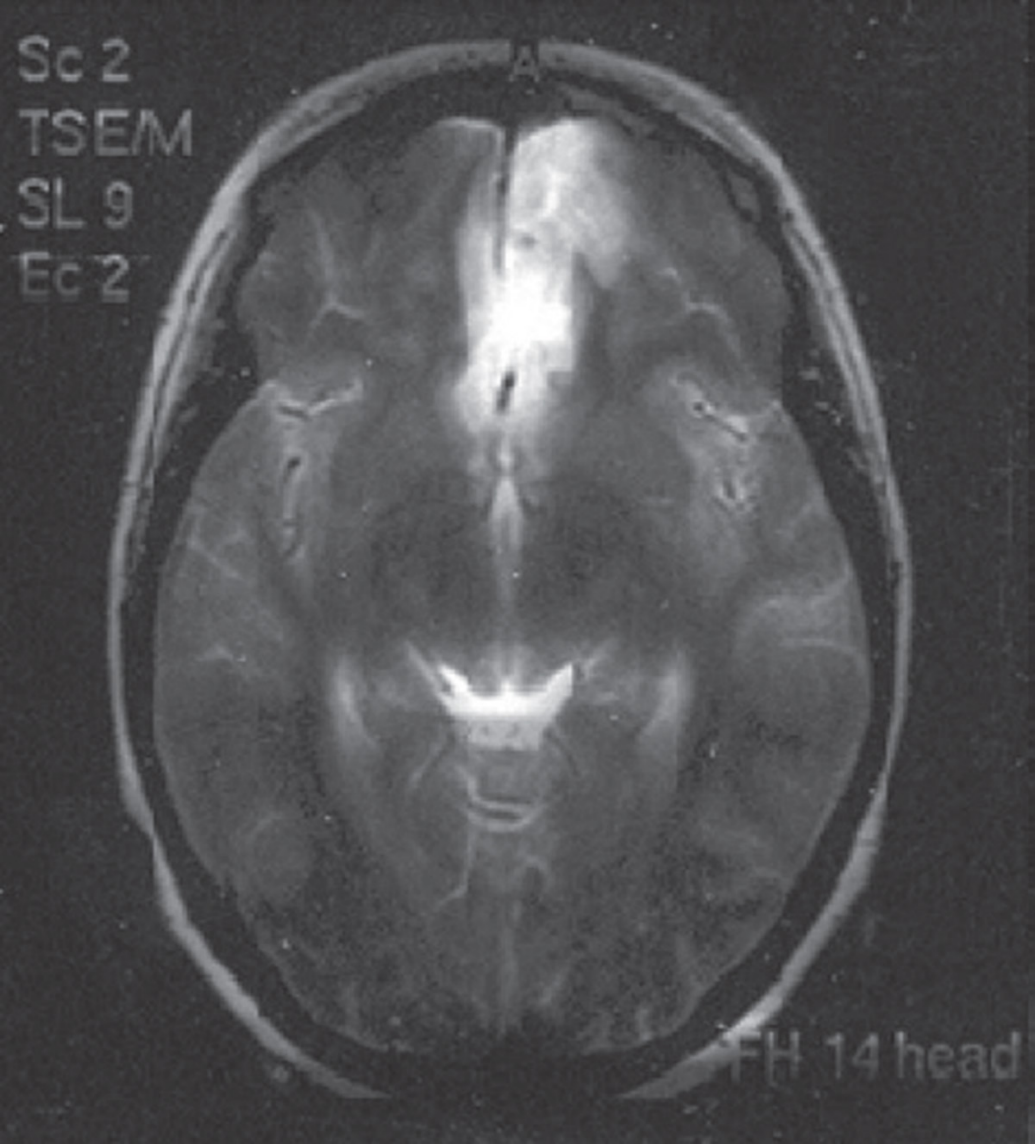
Axial T1, T2, PD, MRI. Diffuse Subarachnoid Hemorrhage; Ischemic Changes in the ACA Territory
